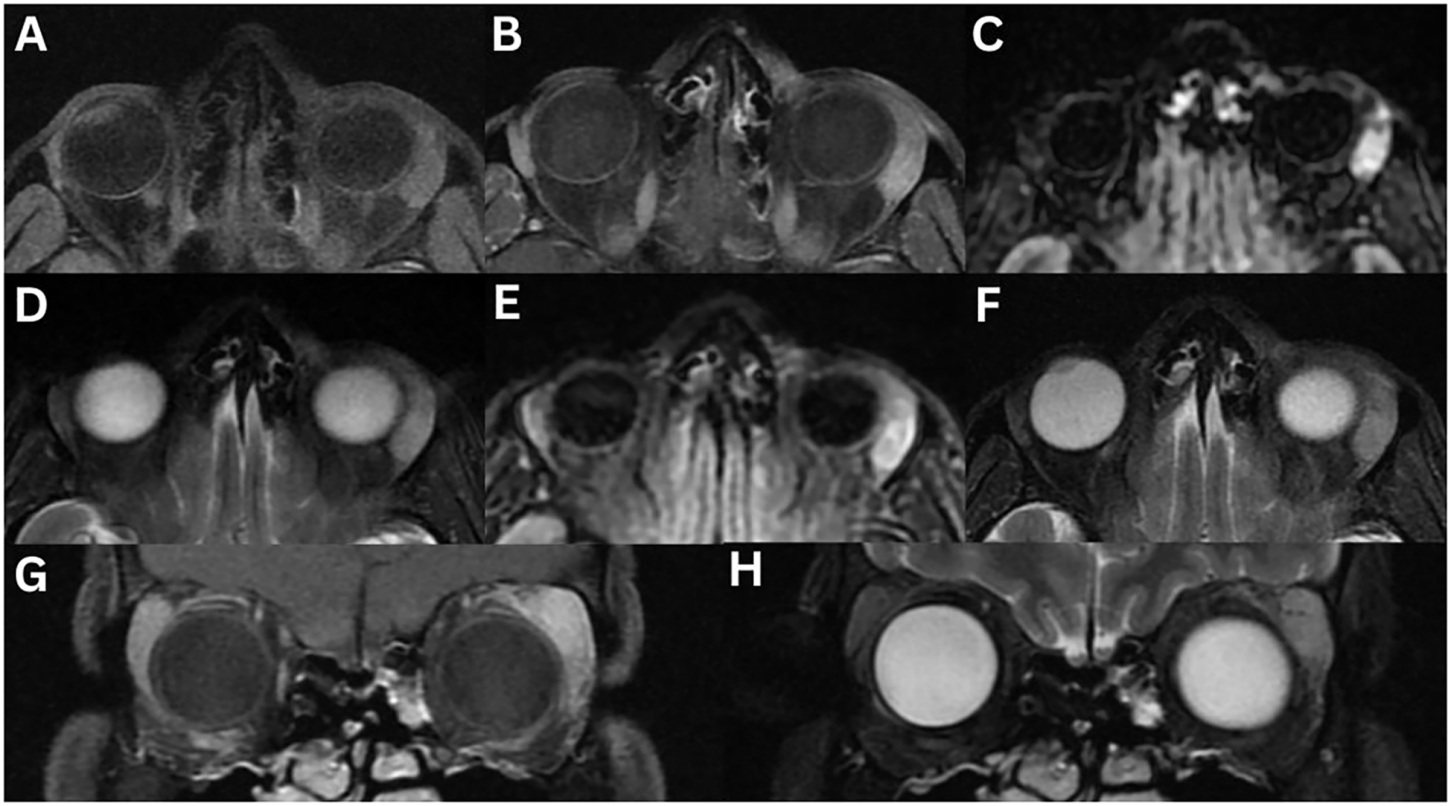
Angiolymphoid hyperplasia with eosinophilia of the left lacrimal gland. MRI T1-weighted axial fat-suppressed FLAIR (A) and axial fat-suppressed contrast-enhanced (B), T2-weighted axial DIR (C), axial (D), axial fat-suppressed FLAIR (E) and axial fat-suppressed (F), T1-weighted coronal fat-suppressed contrast-enhanced (G), and T2-weighted coronal fat-suppressed (H) sequences demonstrate a well-defined, solid, homogeneous mass lesion involving the left lacrimal gland. The mass is hypointense on T1-weighted imaging and hyperintense on T2-weighted and FLAIR sequences, relative to extraocular muscles. There is moderate homogeneous enhancement post-contrast.
